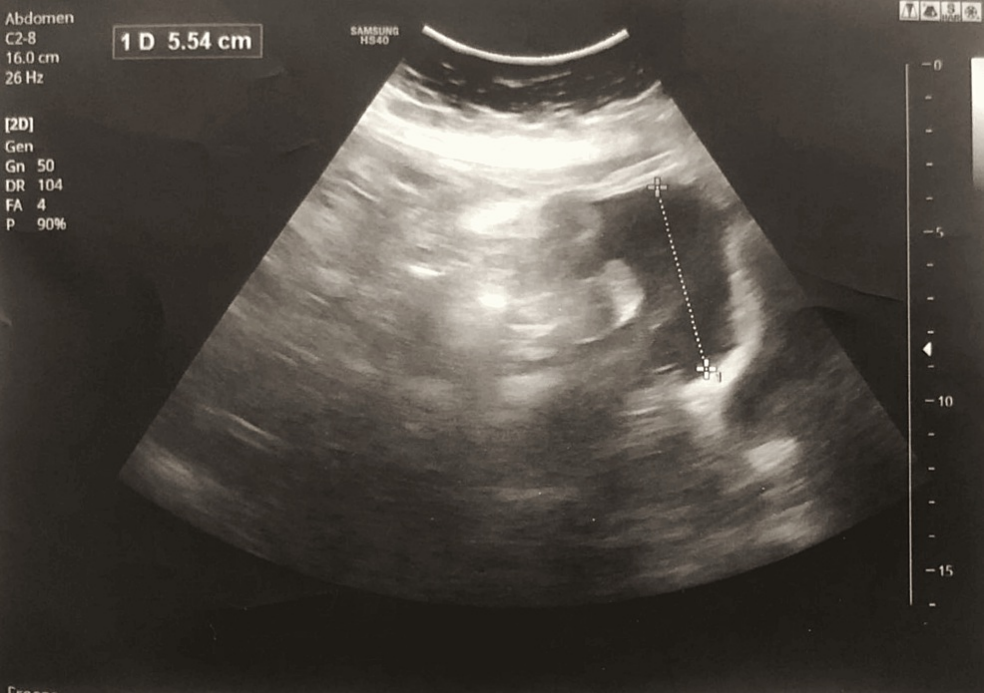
Abdominal ultrasonography
